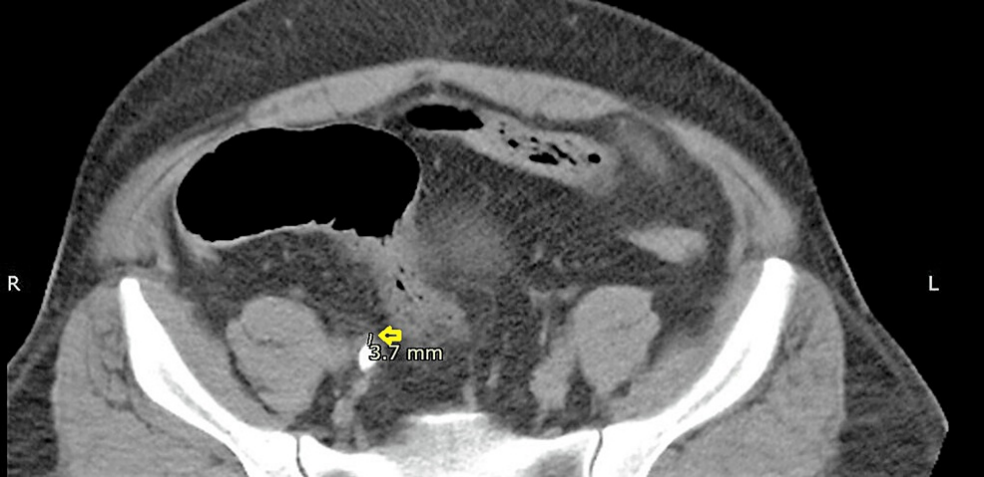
NCCT scan - axial image The image shows a right ureteric stone with a UWT of 3.7 mm. The arrow indicates UWT NCCT: non-contrast computed tomography: UWT: ureteral wall thickness
